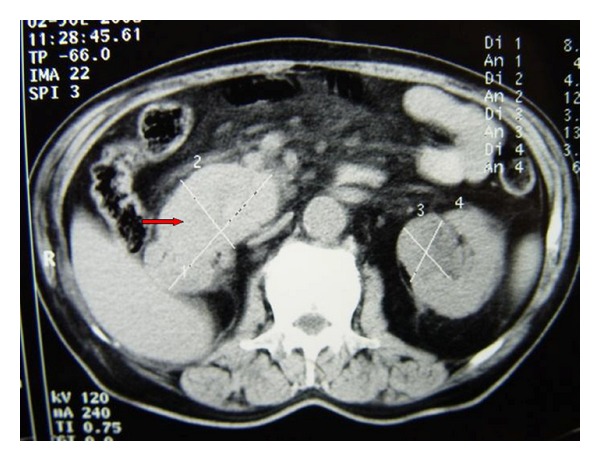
CT examination of the abdomen revealed a mass at the pancreatic head (arrow).
